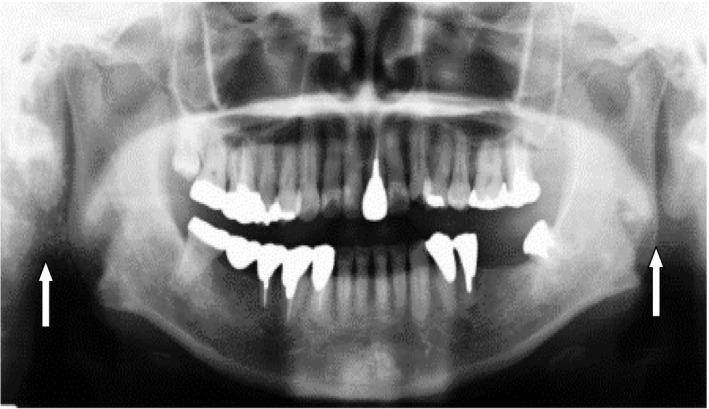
Orthopantomography shows multiple small calcifications around the right gonial angle and fewer calcifications around the left gonial angle
